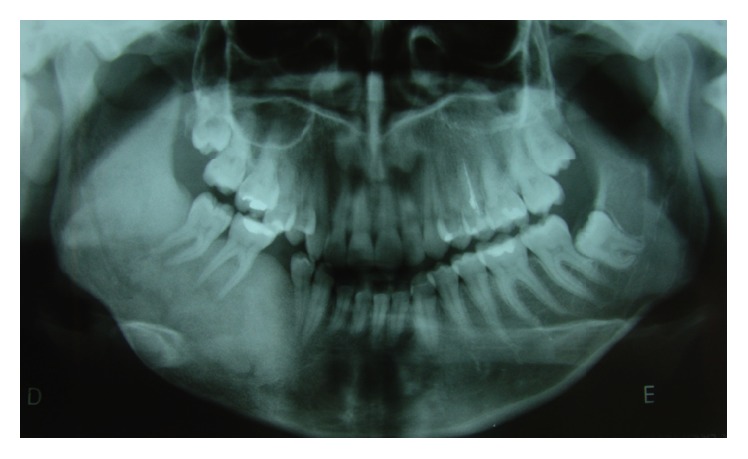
Panoramic X-ray (2009) showing radioopaque area of the image with the appearance of ground glass in the right hemimandible. It can be seen that pieces 4.5 and 4.8 have been removed.
